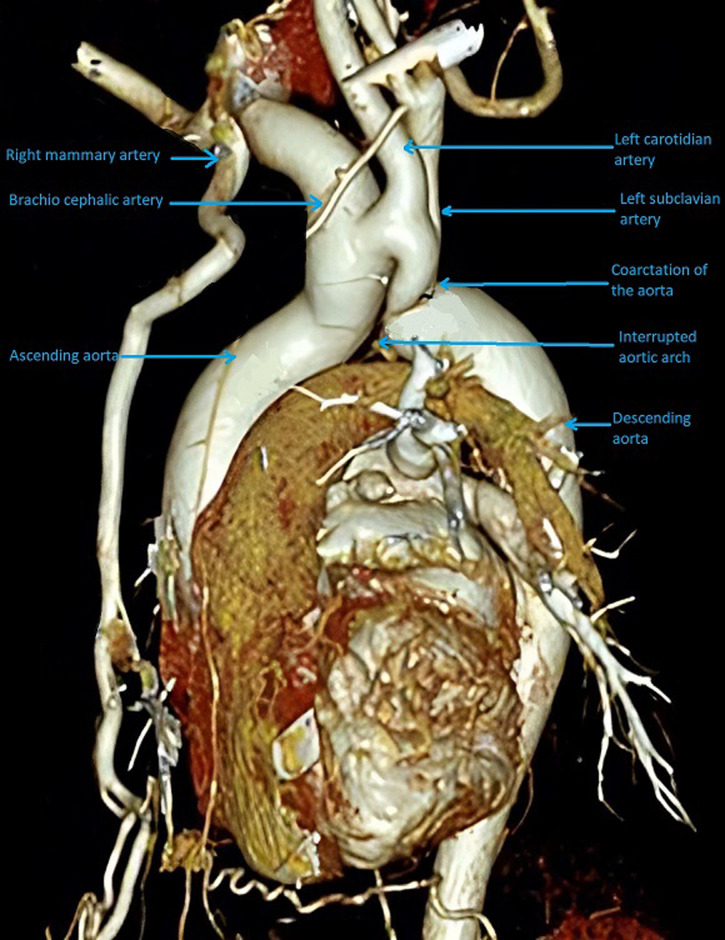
pre-operative computed tomography angiography (CTA) reconstruction
